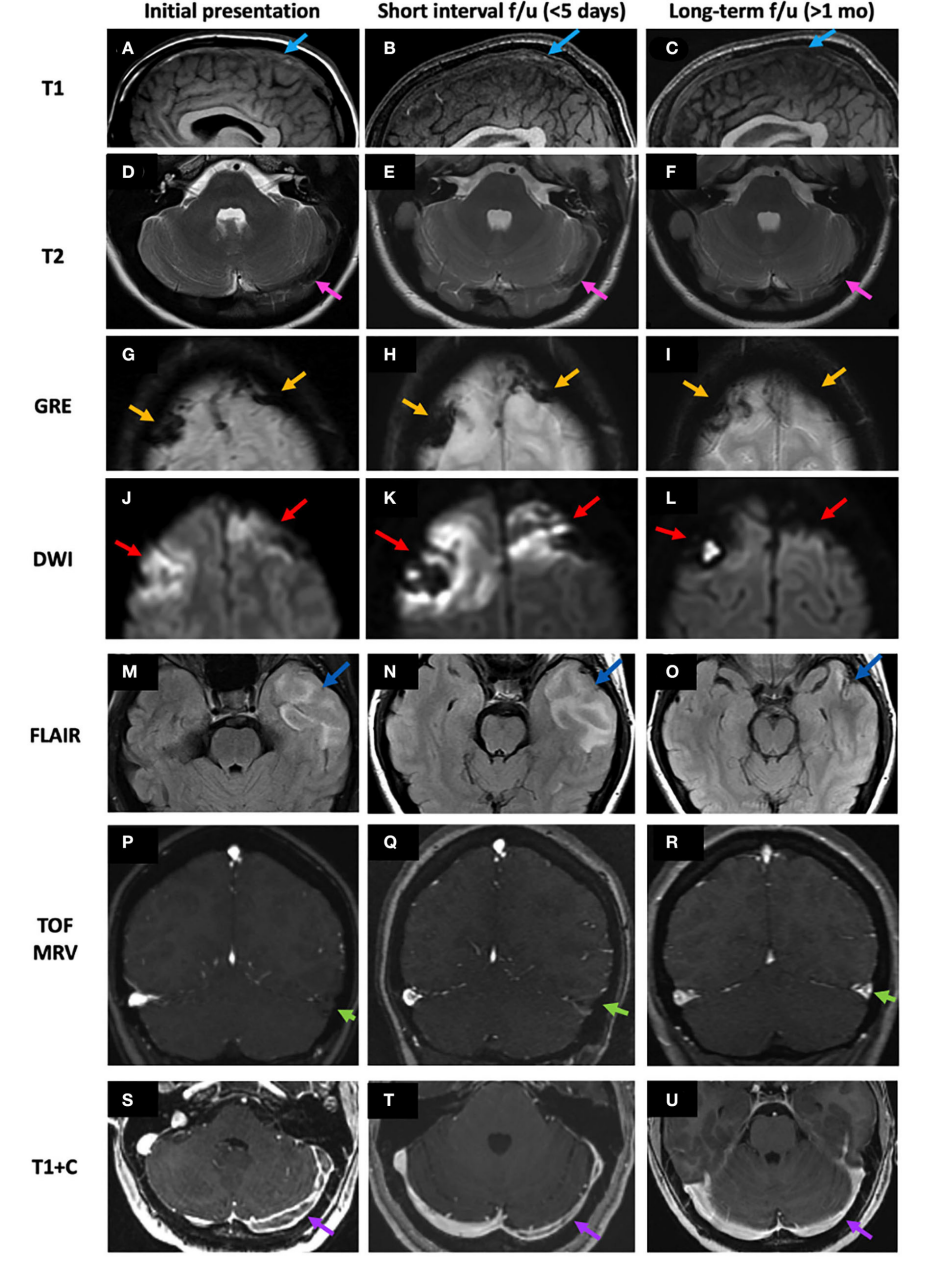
Temporal evolution of CVT on MRI from the acute to late acute to chronic phases. Intrinsic T1 signal within the SSS thrombosis (case 1) progresses from T1 isointense in the acute phase to mixed isointense/mildly hyperintense and to isointense again in the chronic phase (A–C, blue arrows). At the left TS thrombosis (case 3), T2 signal progresses from very hypointense to more isointense and remains mostly isointense (D–F, pink arrows). Bilateral frontal hemorrhagic CVI were seen in a case of SSS thrombosis (case 2). Over time, the bifrontal GRE hypointense signal (G–I, yellow arrows) and corresponding DWI hyperintense signal (J–L, red arrows) both decreased. In a case of left TS and VL infarct (case 3), left temporal FLAIR hyperintensity decreases over time (M–O) as loss of flow-related enhancement resolves at the left TS on TOF MRV (P–R) and the left TS filling defect on T1 + C also resolves (S–U). SSS, superior sagittal sinus; TOF MRV, time-of-flight MRI venography; TS, transverse sinus; VL, vein of Labbé.
